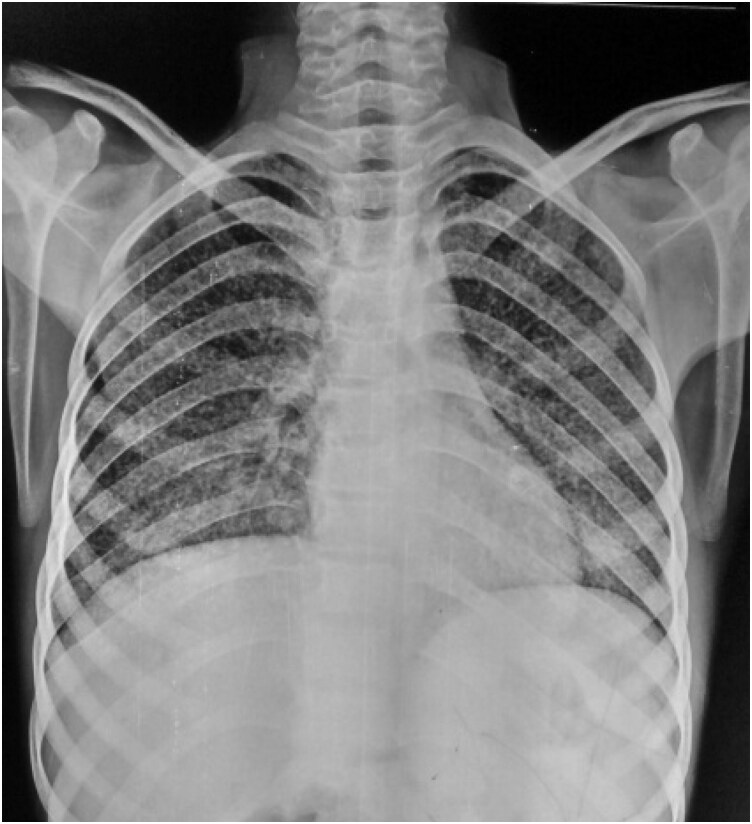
Chest radiograph (posteroanterior view) showing diffuse micronodular opacities throughout both lung fields.
